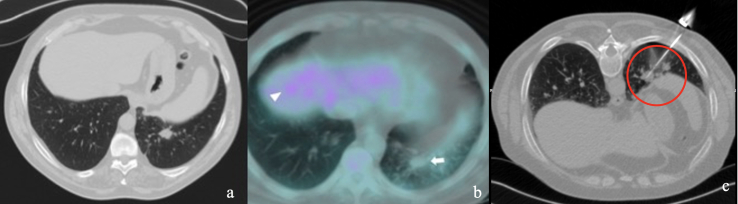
(a) High risk class - preprocedural CT shows a 16 mm solid nodule with basal localization in a 62-year-old patient. (b) ^18^FDG PET-CT of the nodule (white arrow) shows poor metabolic activity (SUVmax 2.4), noticeable when compared to the liver (white arrowhead). According to our scoring system, total points allocated for this lesion were 222 (33 for the patient age; 54 for localization in the lung basis; 57 for small dimension <18 mm; 78 for PET negative result). (c) Biopsy of the nodule shows needle tip at the centre of the target (red circle), yet collected material resulted not diagnostic due to scarcity of the material. ^18^FDG, 18-fludeoxyglucose; PET, positron emission tomography; SUV, standardized uptake value.
